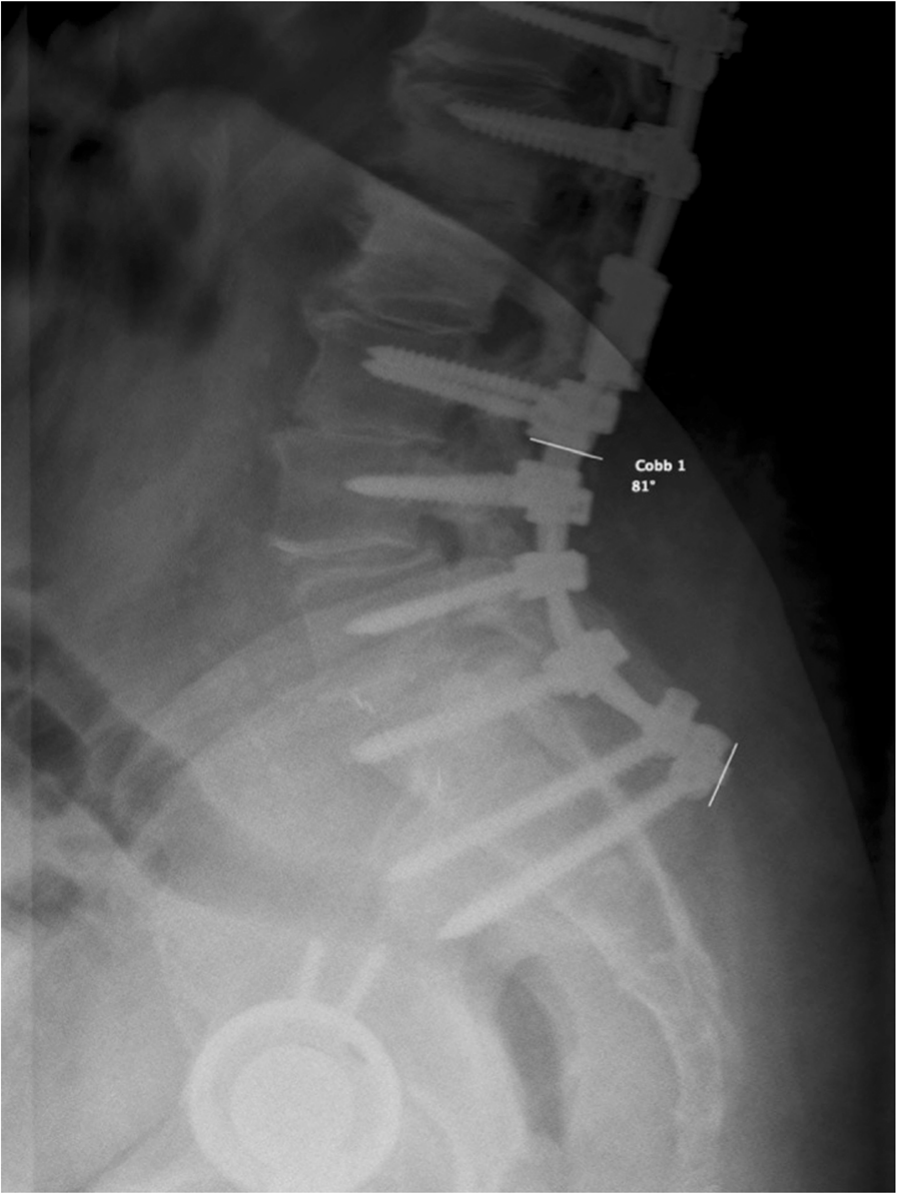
Measuring sagittal rod contour. A Cobb angle was drawn on lateral radiographs incorporating the entire rod curvature. Both lines were drawn perpendicular to the rods
